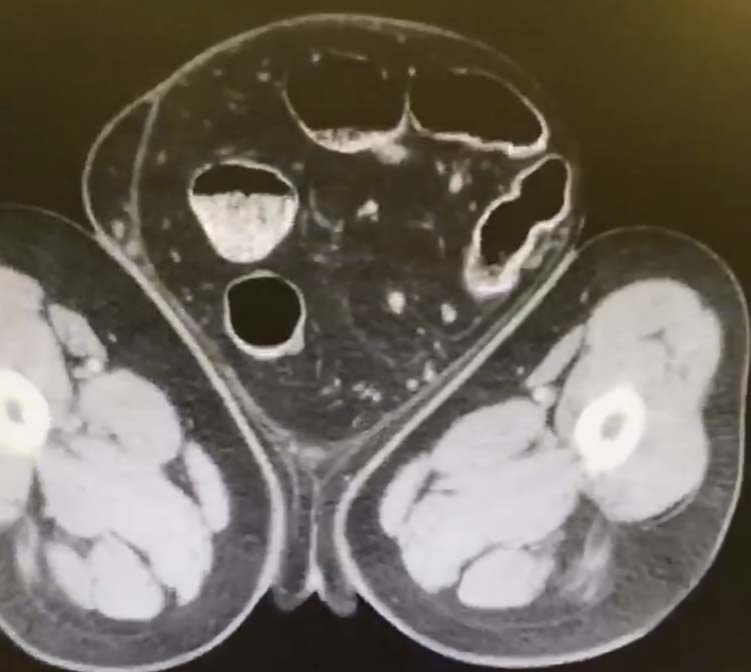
Transverse CT displaying colon within the hernia sac.
